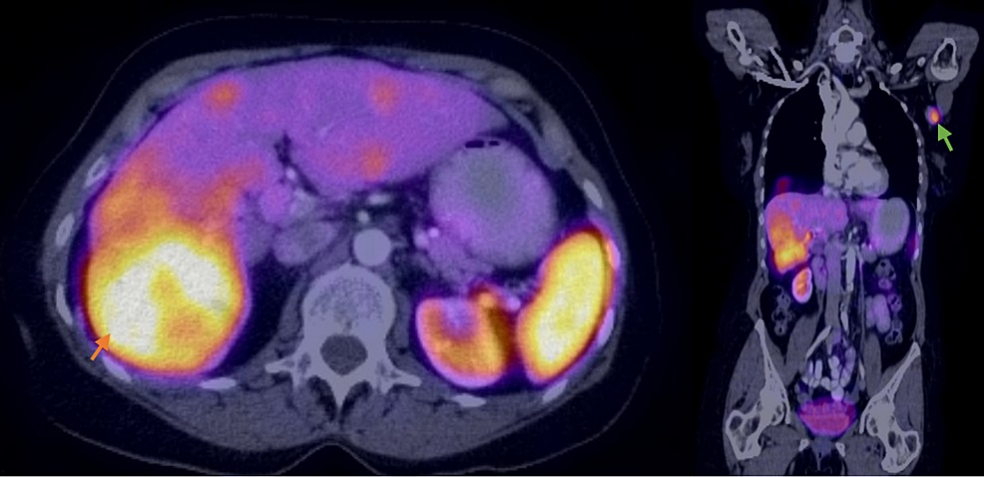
Gallium-68 dotatate PET-CT showing increased radiotracer uptake in liver (orange arrow) and left axillary lymph node (green arrow). PET-CT - Positron emission tomography–computed tomography
